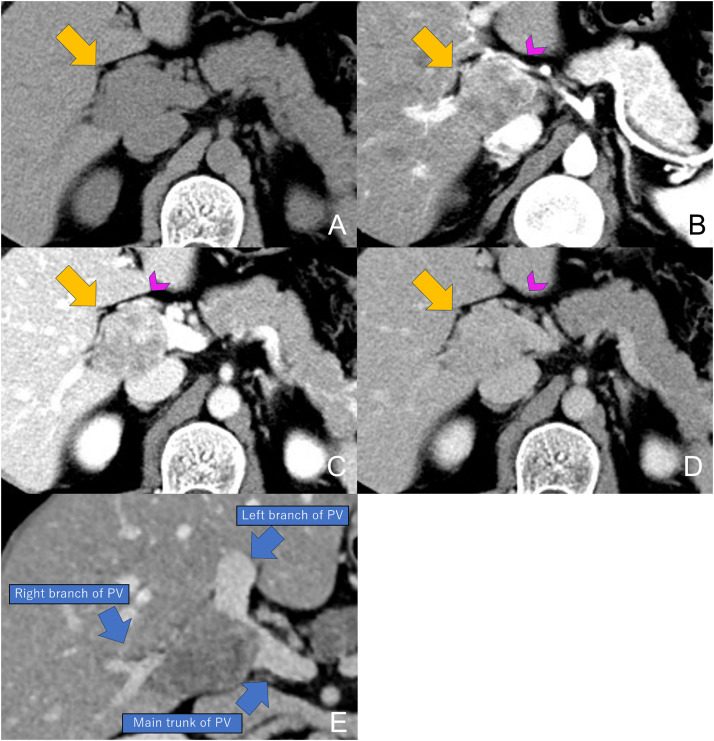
Dynamic CE-CT findings. (A) Unenhanced, (B) arterial, (C) portal venous, and (D) delayed phase images. Unenhanced CT demonstrates a 45-mm low-attenuation mass at the hepatic hilum (yellow arrow). The lesion exhibits heterogeneous and progressively increasing enhancement. Cavernous transformation of the portal vein at the hepatic hilum is also observed (pink arrowheads). The reconstructed view (E) clearly demonstrates the fusiform intraluminal configuration of the tumor and precisely depicts its anatomical relationship to the main portal trunk and the right and left portal branches (blue arrows). PV: portal vein.
